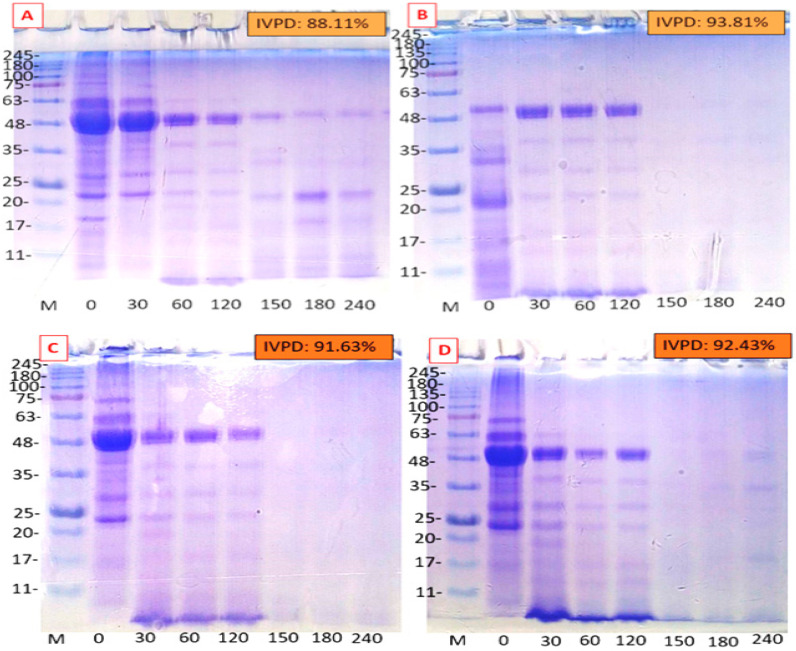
Influence of extraction technique on in vitro protein digestibility pattern of MSPI. (A): Alkaline extraction, (B): enzyme-assisted extraction, (C): ultrasonic-assisted extraction, (D): microwave-assisted extraction. IVPD: in vitro protein digestibility. Gel used: 12% separating gel and 4% stacking gel. 15 μg protein was loaded in each lane.
